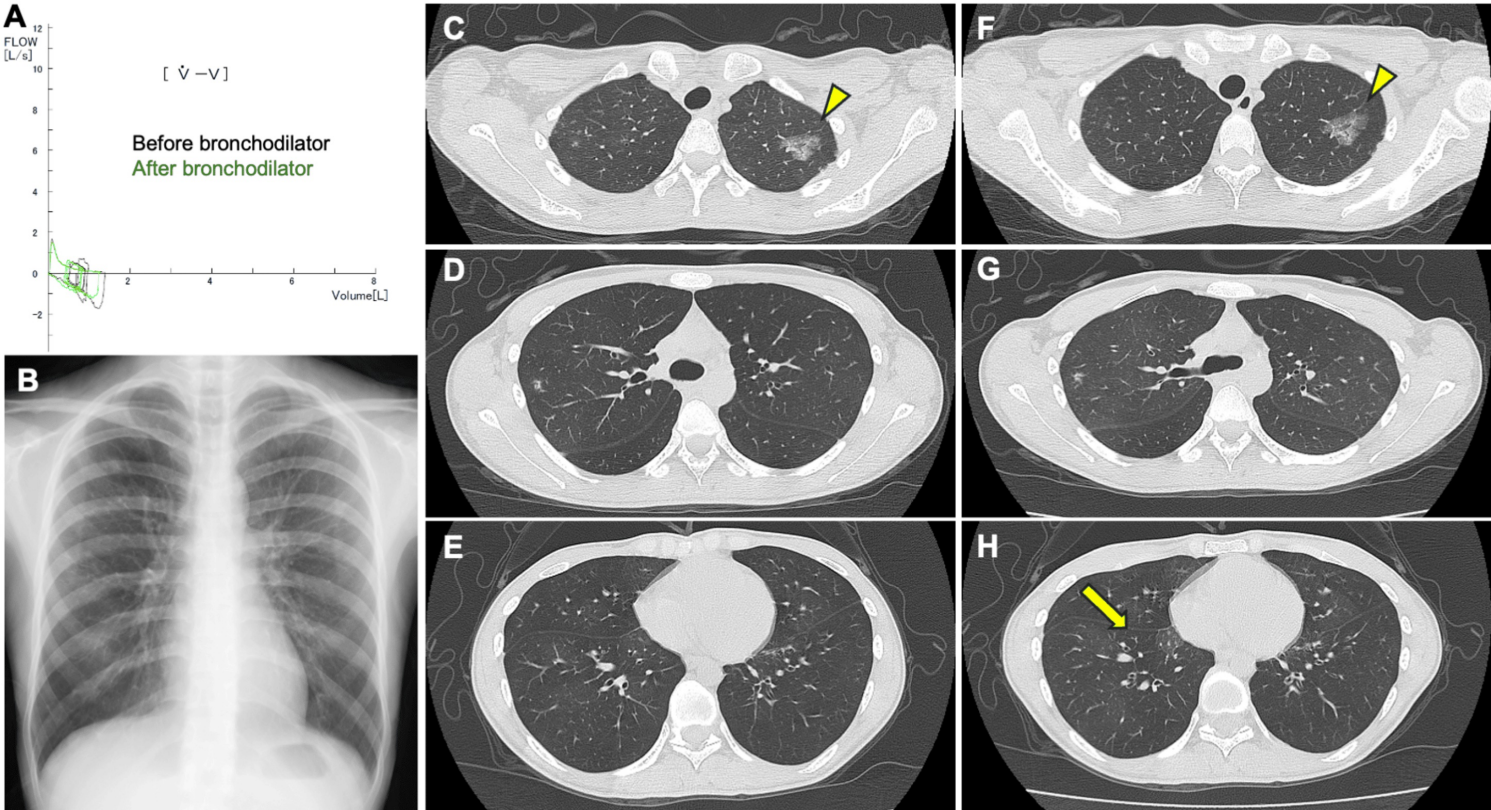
Pulmonary imaging and spirometry findings (A) Flow–volume curves before and after bronchodilator administration. (B) Chest X-ray imaging of the patient at initial presentation. (C–E) Initial CT showing focal ground-glass opacities (arrowheads) and small nodules predominantly in the bilateral upper lobes. (F–H) Expiratory CT images demonstrating areas of air trapping (an arrow) especially in the bilateral lower lobes.
